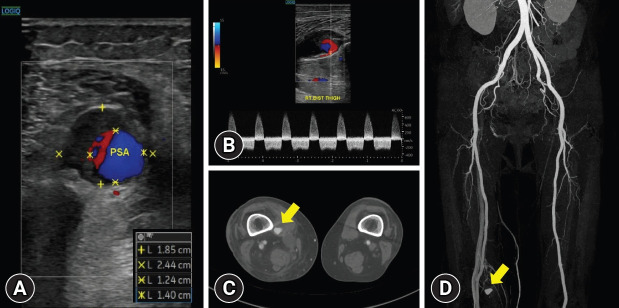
Preprocedural duplex ultrasonography and computed tomography. (A) Swelling is observed in the right lower extremity. A pseudoaneurysm (PSA) is identified in the right distal thigh. (B) Color Doppler imaging showing the “yin–yang sign” within the pseudoaneurysm sac, and a characteristic “to-and-fro” flow pattern is observed at the neck. (C) Axial computed tomography image showing the pseudoaneurysm (arrow). (D) Computed tomography angiography demonstrating the pseudoaneurysm (arrow).
